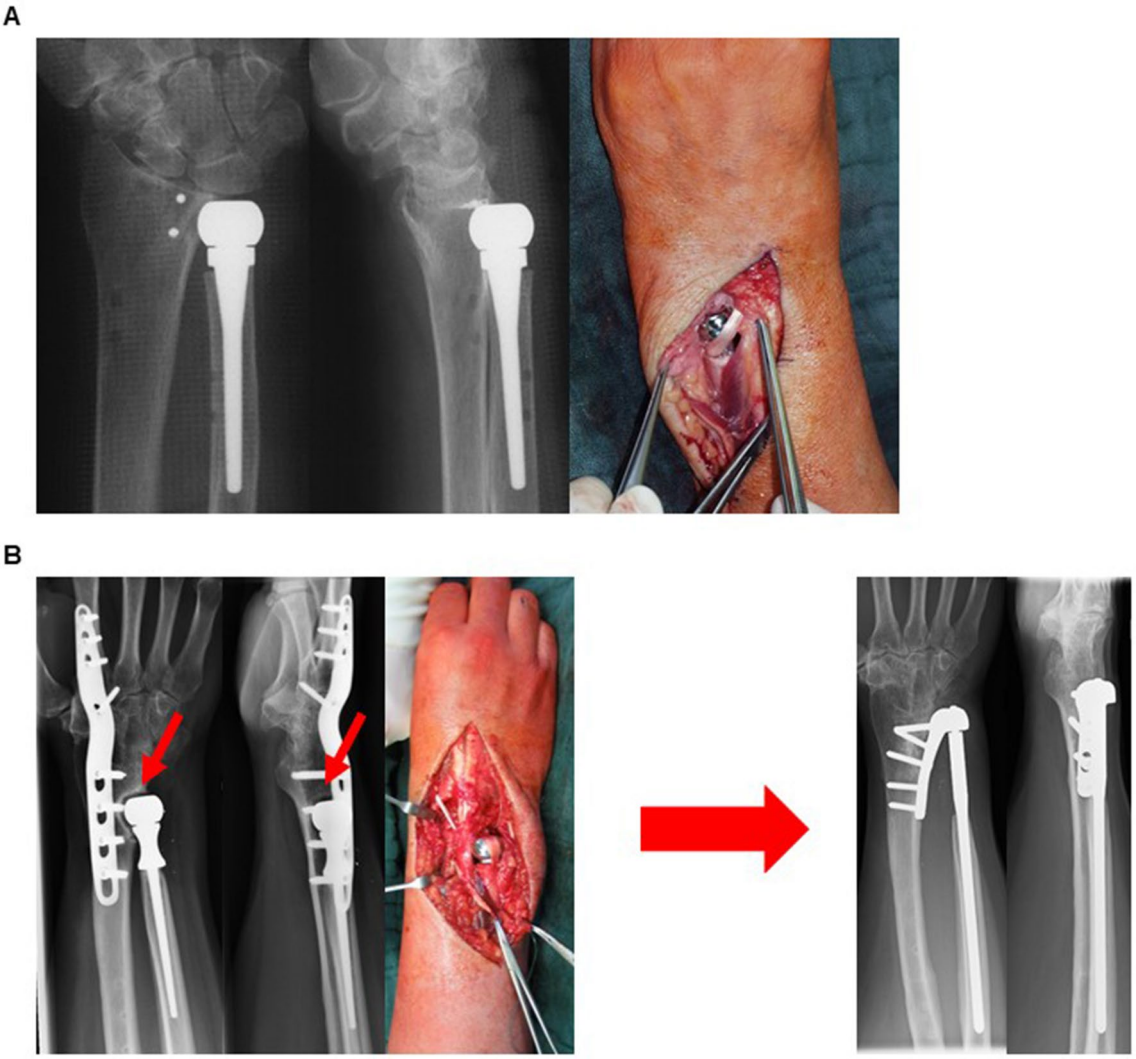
Radiograph of an ulna head prosthesis with intraoperative pictures of the ligament reconstruction using a tendon graft to replace the lost stabilizers. Radiograph of an ulna head prosthesis 3 years after implantation significantly gouging into the distal radius causing pain and instability, on the right side condition after implantation of an Aptis DRUJ prosthesis
